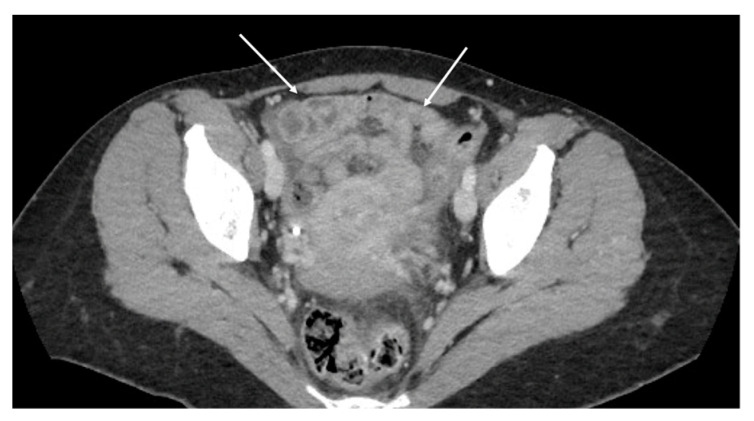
Contrast-enhanced CT scan, axial view. Case of theca pattern. Nodular thickening of the peritoneum lining the loops of the small bowel in pelvis (white arrows).
